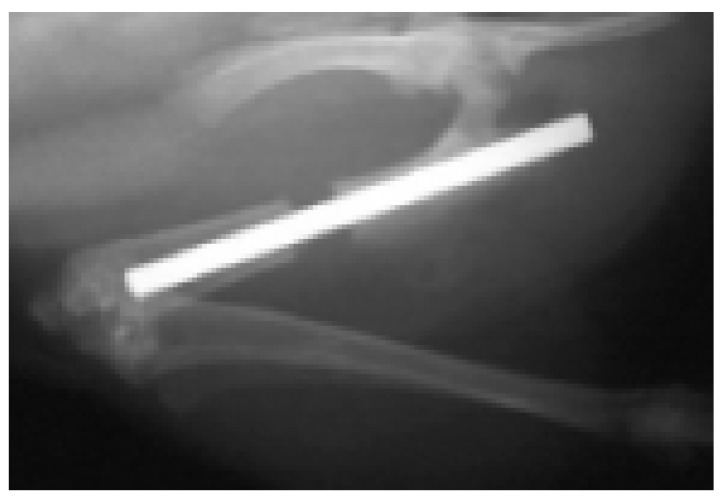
Radiograph of left femoral bone just after osteotomy and BMG intramedullary nail implantation.
